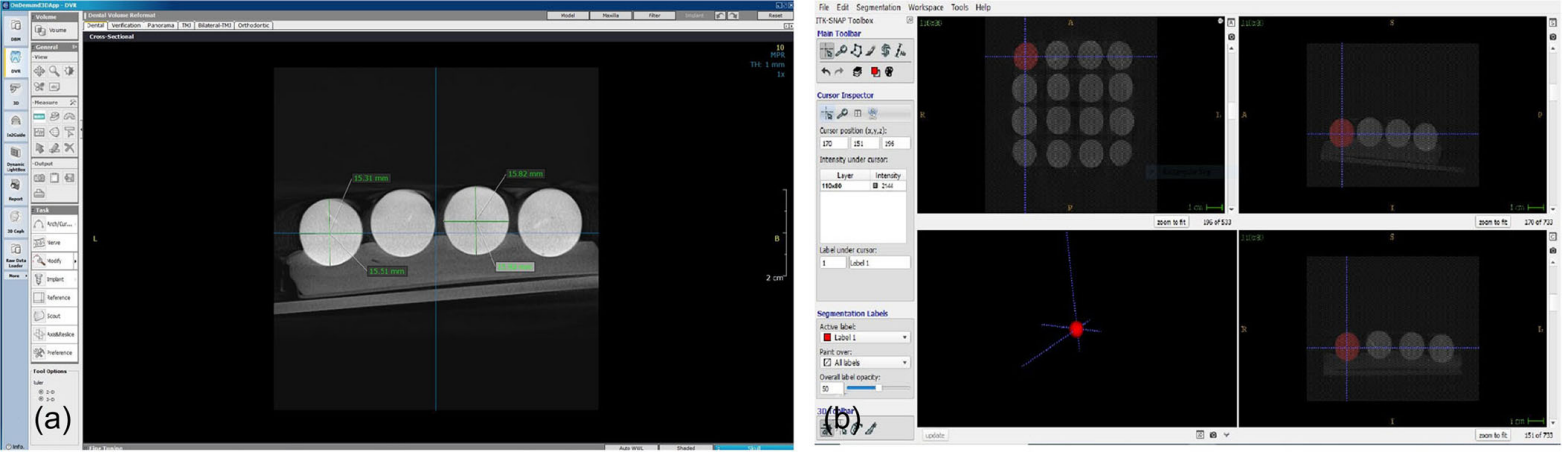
(a) Measurement of horizontal and vertical dimensions in CBCT axial sections in ondemand software. (b) Measurement of ball volume in itk‐snap software. CBCT, Cone‐beam computed tomography.
